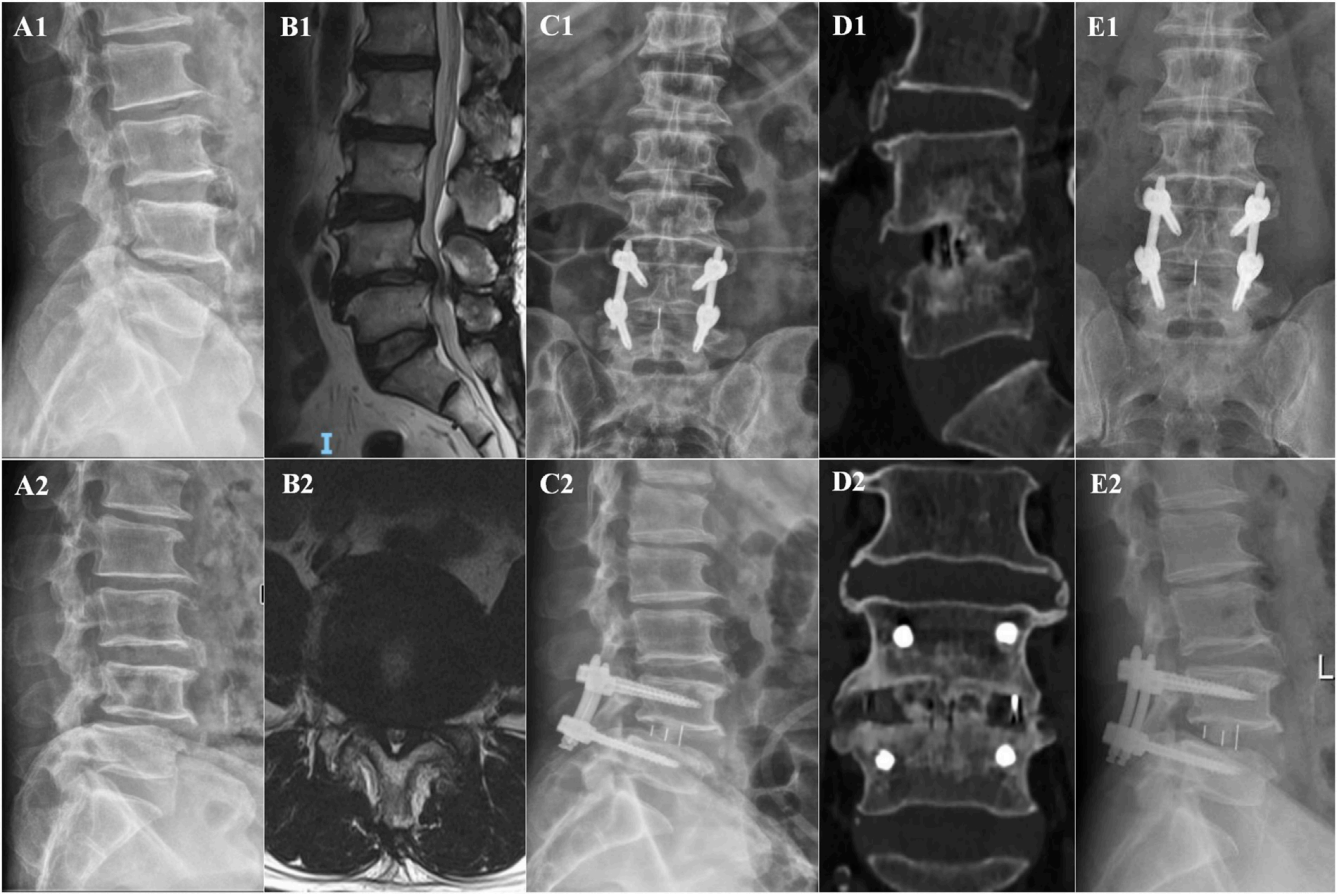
The radiological images of OLIF-PIFPS typical case. (A1,A2) Preoperative dynamic X-ray films; (B1,B2) Preoperative sagittal and axial MR images; (C1,C2) A−P, lateral X-ray film at 1 day postoperatively; (D1,D2) Sagittal and coronal CT images at 12 months postoperatively; (E1,E2) A−P, lateral X-ray film at 12 months postoperatively.
